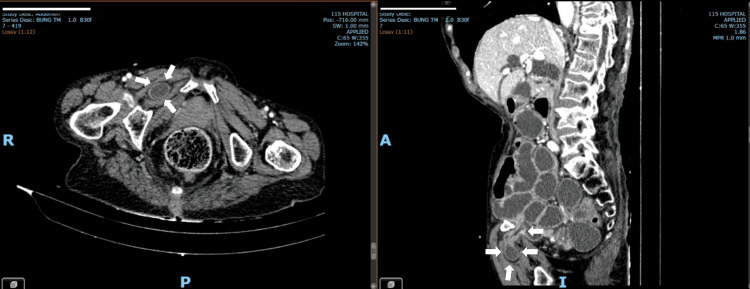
Images of the obturator hernia Axial and sagittal CT scans after contrast injection revealed intestinal obstruction at the transition point in the right iliac fossa due to the obturator hernia (the arrows).
